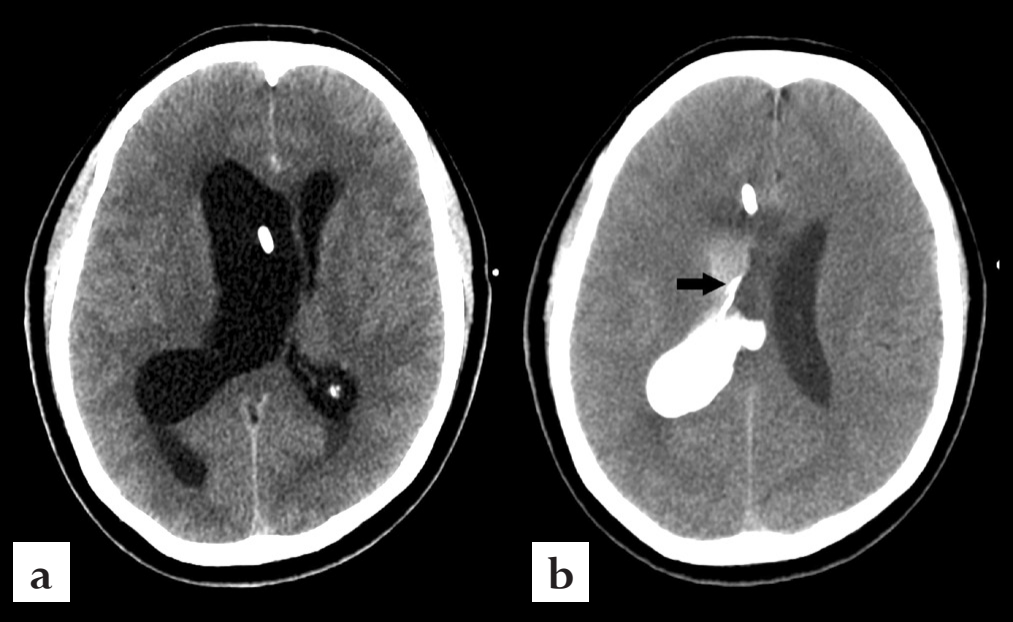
Case # 2. (a): Axial CT scan of the brain without contrast showing right ventriculostomy catheter in the lateral ventricle. (b): Axial CT scan of the brain with ventricular injection of iohexol showing the outline of the right ventricular lesion.
